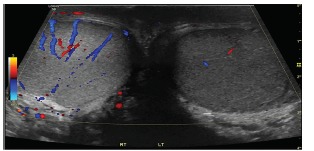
Formal ultrasound of both testes with demonstration of decreased Doppler vascular flow on left testicle compared to right.
